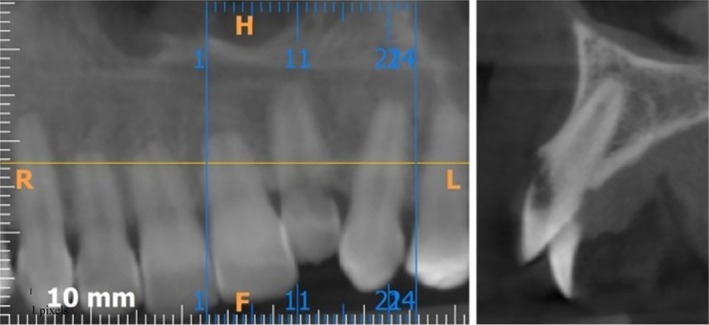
CBCT imaging confirmed cervical resorption of the upper left lateral incisor.
